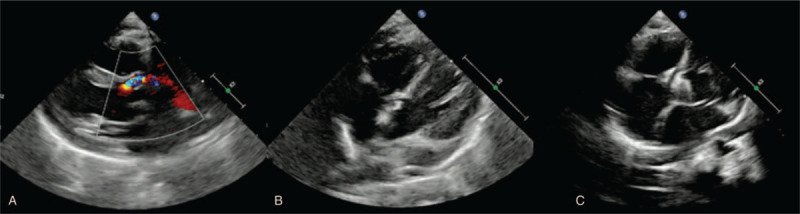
Echocardiography before and after closure of LV-RA shunt. (A) For the blood flow from left ventricle shunt into right atrium. (B) Shows a narrow tunnel for the defect. (C) Demonstrates the position of Amplatzer duct occluder II after the closure. LV-RA = left ventricle-right atrium.
